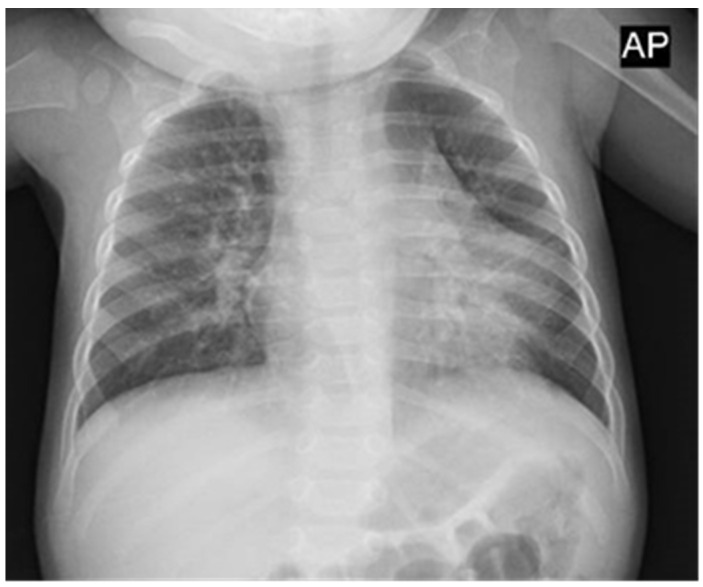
Chest X-rays: diffuse bilateral peribronchial thickening in the hilar and perihilar regions with alveolar confluence in the left perihilar inferior area. AP: anteroposterior.
